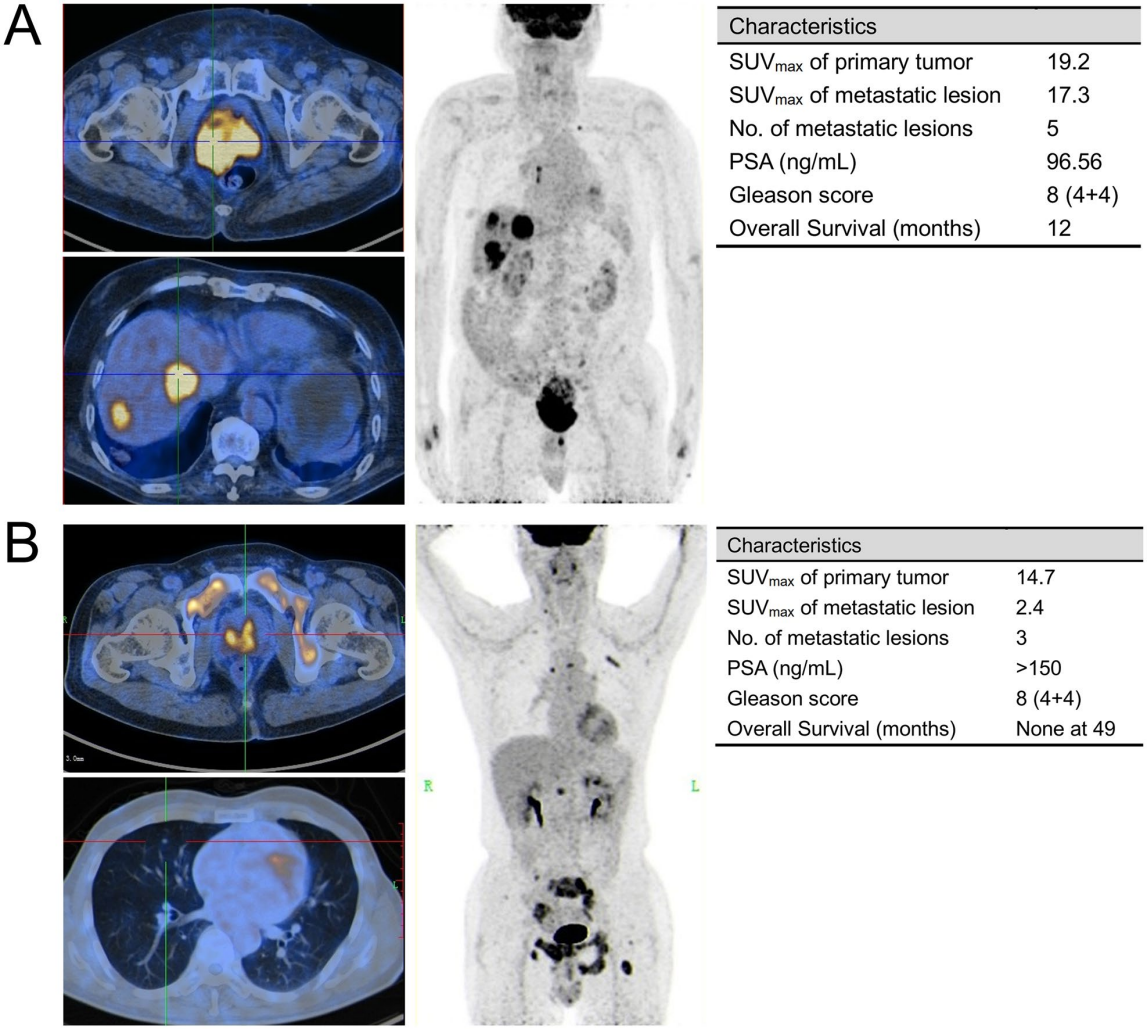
Two representative cases of mHSPC patients with similar baseline characteristics but distinct prognoses. (A) An 85-year-old man who was first diagnosed with liver metastatic prostate cancer in 2020, with Gleason score of 4 + 4 = 8 and serum PSA of 96.56 ng/ml. [^18^F]FDG PET/CT (upper left: axial PET/CT imaging of primary tumor; lower left: axial PET/CT imaging of liver metastatic lesion; Right: maximum intensity projection (MIP)) showed widespread visceral and lymph node metastasis. Although treated with endocrine therapy and chemotherapy immediately after diagnosis, he had a dismal prognosis, with an OS of 12 months from definitive PET/CT diagnosis to death; (B) a 67-year-old man who was first diagnosed with lung metastatic prostate cancer in 2018, with Gleason score of 4 + 4 = 8 and serum PSA of >150ng/ml. [^18^F]FDG PET/CT (upper left: axial PET/CT imaging of primary tumor; lower left: axial PET/CT imaging of lung metastatic lesion; right: maximum intensity projection (MIP)) showed multiple visceral and bone metastasis. This patient received ADT (including surgical castration) after being diagnosed. No evidence of tumor progression was noted with follow-up as of November 2022.
